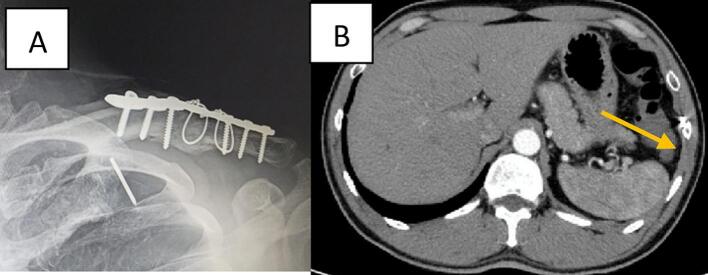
(A) Post-operative X-ray. (B) CT – Scan evaluation show the wire position below 9th costae with no organ damage.
